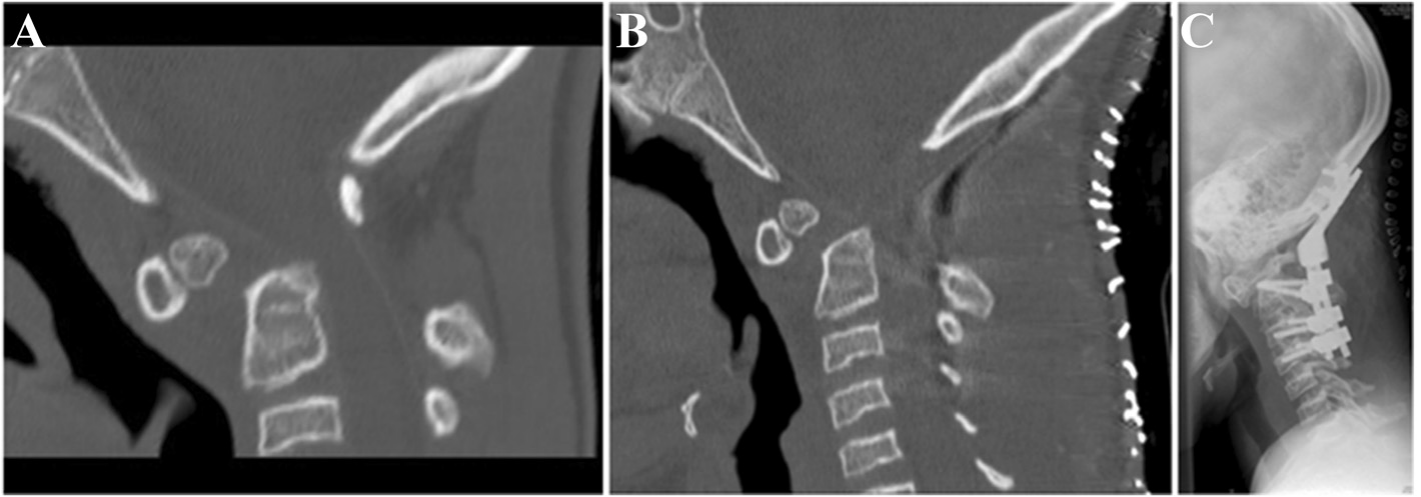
Radiological images from Case 1. a) Pre-operative sagittal midline CT (on bone windows) shows an os odontoideum widely separated from the body of C2 with a widening of the anterior atlanto-dental distance. There is a marked reduction in the sagittal bony canal dimensions between C2 body and the C1 posterior arch) b) Post-operative sagittal midline CT (on bone windows) demonstrates resection of the posterior arch of atlas and some reduction of the os odontoideum with a reduced distance between the os and the body of C2. c) Lateral plain radiograph demonstrates the occipito-cervical rod and screw fixation
